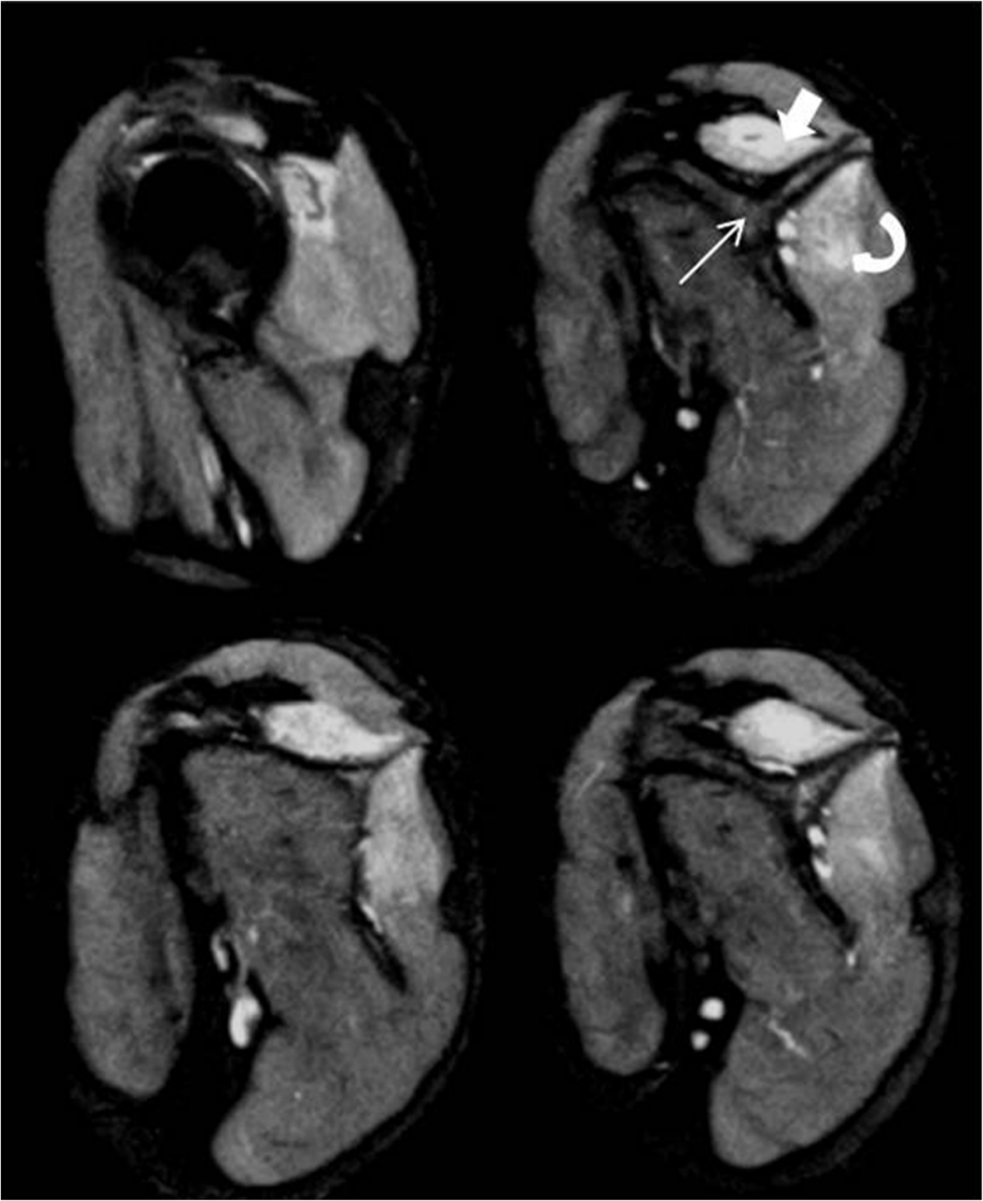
Magnetic resonance imaging of patient 1 at the level of shoulder girdle including the periscapular muscles. Sagittal T2-weighted fat-suppressed contiguous section at the level of shoulder showing edema in suprasinatus (solid arrow) and infraspinatus (curved arrow) muscles, respectively above and below the spine of scapula (straight arrow). The brachial plexus in this patient, however did not reveal any abnormality.
